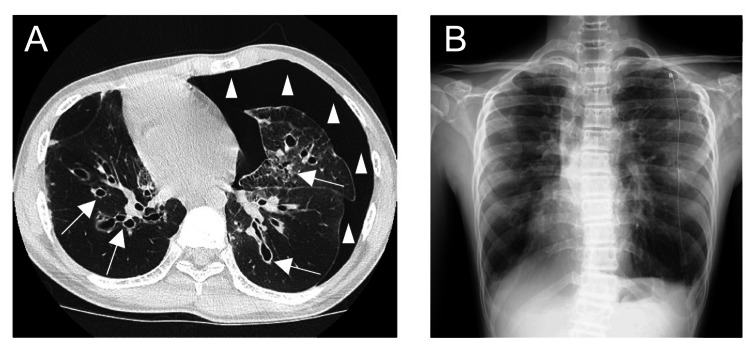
Computed tomography and chest radiograph These two images show that the right-sided deviation of the mediastinum is due to a left pneumothorax. (A) High-resolution computed tomography image showing hyperinflation, emphysematous changes, bronchiolitis obliterans (white arrows), and pneumothorax (white triangles). (B) Chest radiograph taken the day before surgery after insertion of a chest drain.
